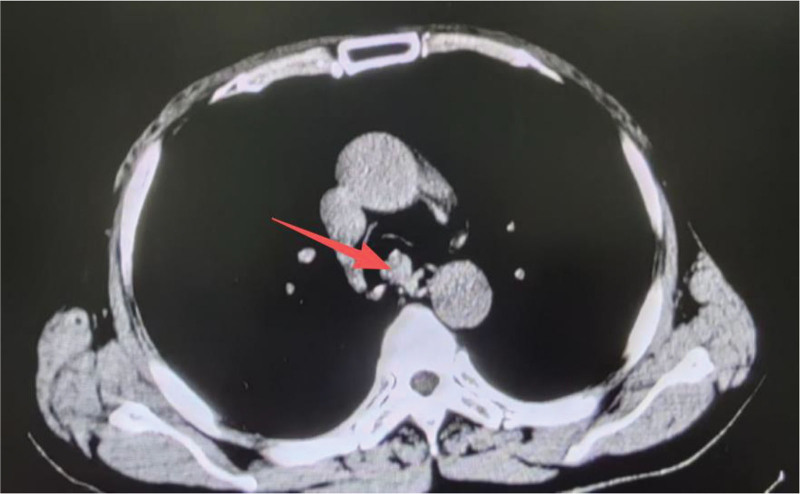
The tracheal tumor depicted on computed tomography, indicated by the red arrow.
